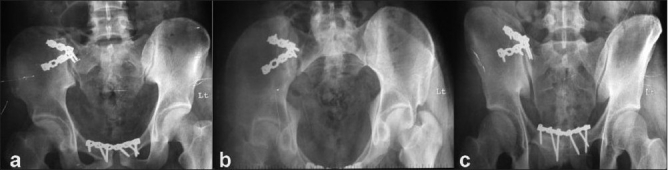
Postoperative anteroposterior (a), inlet (b), and outlet (c) radiographs showing reduction and fixation of the pelvic disruption. Note the divergent nature of the sacroiliac reconstruction plates to maximize the stability of fixation. Also, note the anterior symphyseal fixation which closes the pelvic ring
